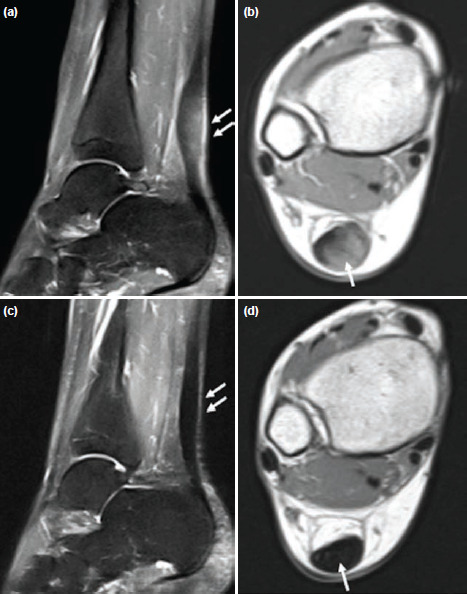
(a) Sagittal short tau inversion recovery (STIR) MR image and (b) axial T2W MR image of the right ankle showing non-insertional Achilles tendinosis with fusiform thickening and hyperintense signal of the Achilles tendon (white arrows). (c) Sagittal STIR MR image and (d) axial T2W MR image showing the Achilles tendon resuming its normal signal intensity and appearing less thickened compared to previous study (white arrows) at six months following multiple needling and a five-weekly injections of PBSCs plus HA.
